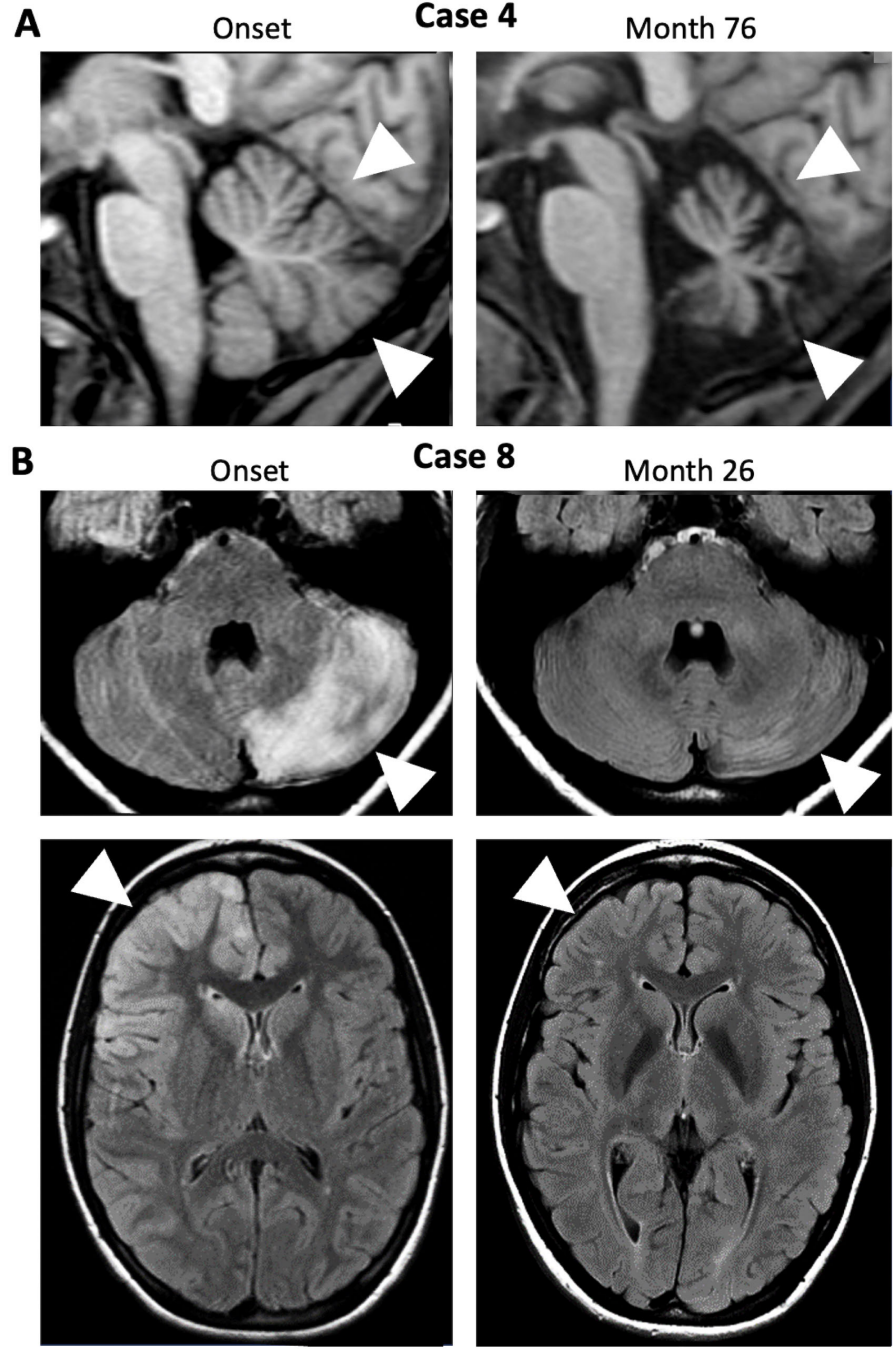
MRI changes in NMDAR-E patients with cerebellar ataxia are rare. (A) Sagittal 1-weighted MRI of Case 4 at onset (left panel) and after recovery from severe NMDAR- E but with persisting bilateral cerebellar ataxia (month 76; arrows indicate cerebellar atrophy). (B) FLAIR images of Case 8 with reversible left-sided hemiataxia in the acute phase (onset, left panels) show diffuse, mostly cortical left cerebellar T2-hyperintensity (upper left panel, arrowhead) possibly resulting from cerebellar diaschisis following right frontal status epilepticus associated with right frontal cortical hyperintensities (lower panel, arrowhead). T2-hyperintensities were reversible (Month 26, right panels.
